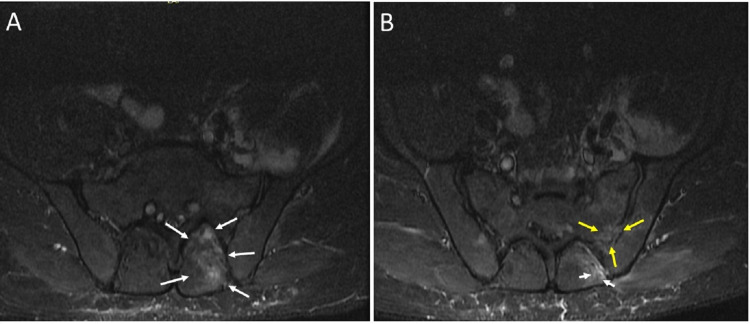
Magnetic resonance imaging (MRI) scan of the lumbosacral spine demonstrating inflammatory changes. (A) Pyomyositis of the left-sided erector spinae muscle (white arrows), and (B) pyomyositis of the left iliacus muscle (yellow arrows) and erector spinae muscle (white arrows).
